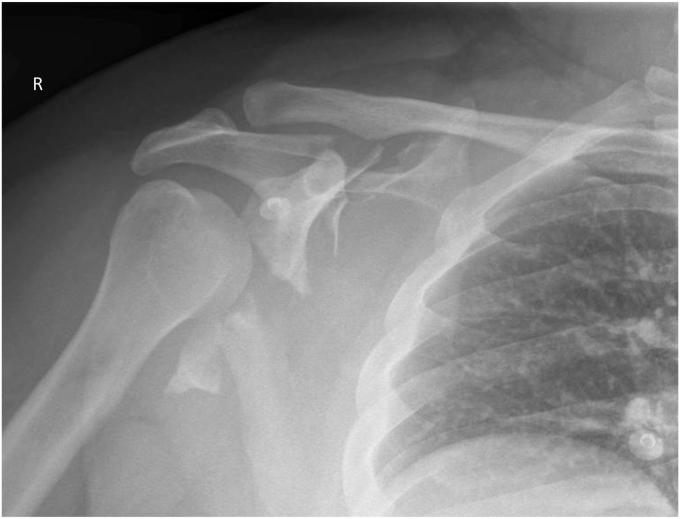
Initial plain radiographs.
